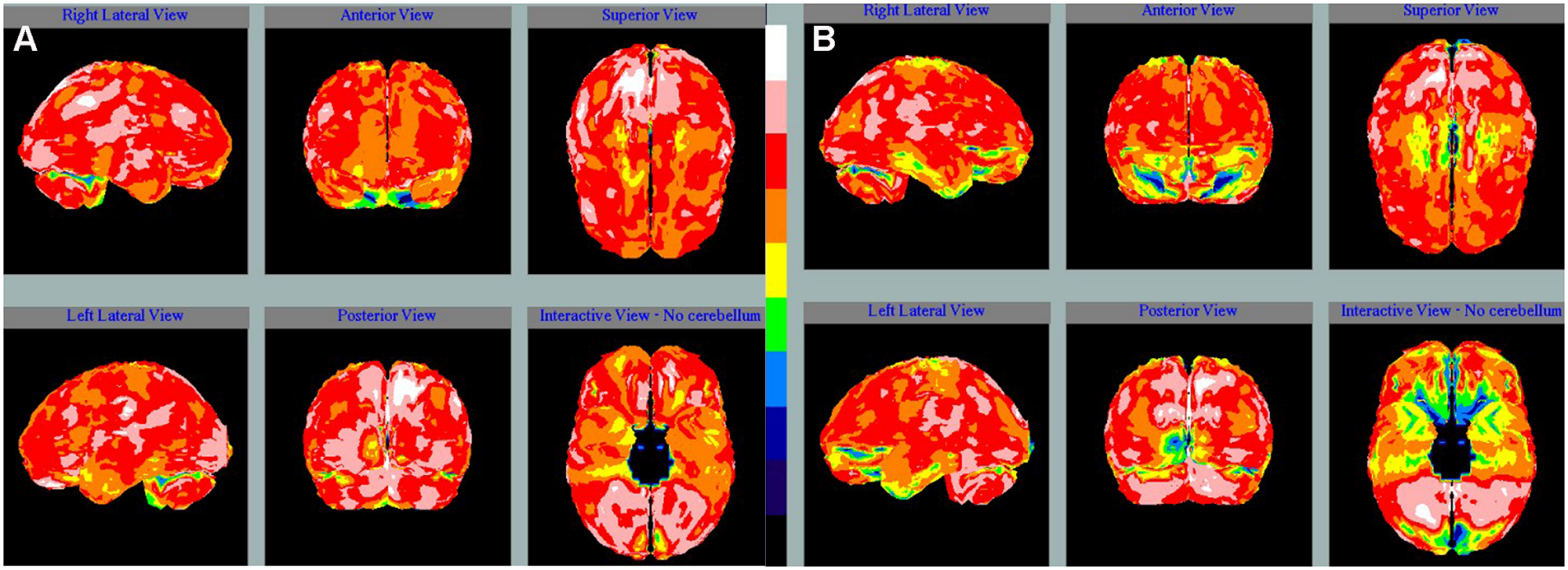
The images of Tc-99m ECD brain SPECT of two patients. (A) A 50-year-old female had occasional dizziness for one year. Brain MRI did not reveal any definite organic brain change. The autoimmune screen showed no positive autoantibody including antiphospholipid antibody. The Tc-99m ECD SPECT demonstrated only small foci of hypoperfusion at bilateral hemispheres with the coefficient of variation as 10.8%. (B) A 39-year-old female had initial presentations of depressive mood and seizure. She had positive ACA IgG, APhL IgG, anti-β2GPI IgG and lupus anticoagulants. There was no definite organic brain change in the brain MRI; however, the Tc-99m ECD SPECT revealed large areas of hypoperfusion at bilateral hemispheres with the coefficient of variation up to 20.9%. Ten months later, she developed an ischemic stroke.
